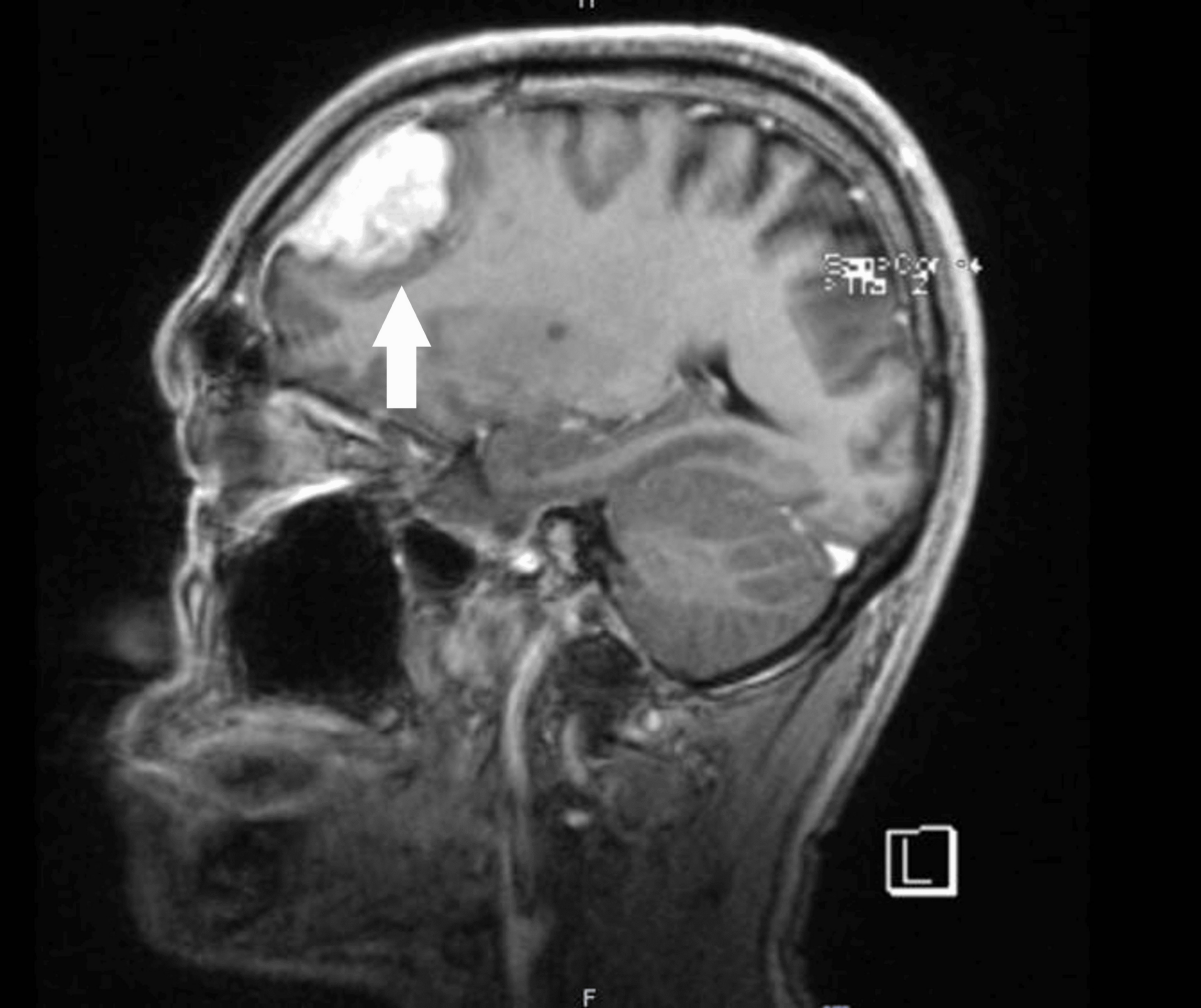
Sagittal-view T1-weighted image showing a left frontal lobe lesion
